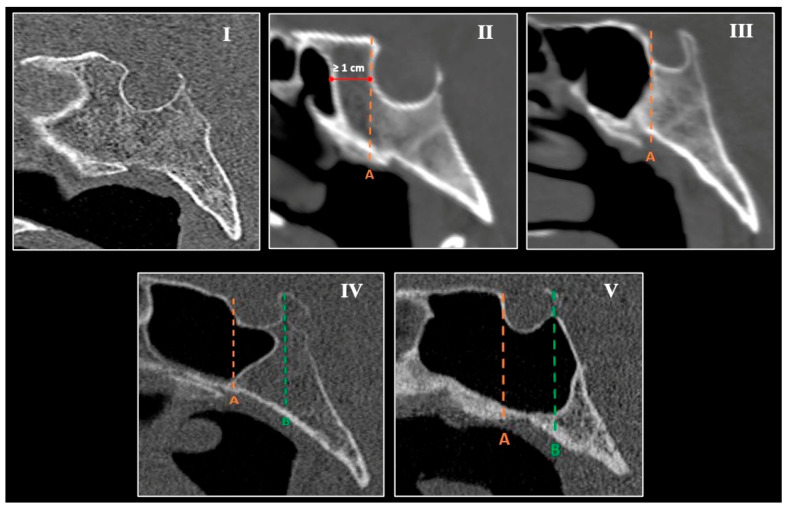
Main types of sphenoid sinus pneumatization (SSP) on midsagittal computed tomography (CT) images. Representative midsagittal CT sections demonstrating the five principal morphologic variants of the sphenoid sinus (SS) based on the relationship of pneumatization to the sella: (I) agenesis—complete absence of sinus cavity; (II) conchal type—minimal pneumatization not extending to the anterior wall of the sella (≥1 cm anterior to it); this case derived from the excluded population and it was not observed in the current sample; (III) presellar type—pneumatization reaches but does not pass the anterior wall of the sella; (IV) sellar incomplete type—pneumatization extends to the posterior clinoid process, involving both the anterior wall and sellar floor; (V) sellar complete type—pneumatization extends beyond the posterior wall of the sella into the basilar portion of the occipital bone. Dotted orange line (A) = vertical coronal plane through the anterior sellar wall; dotted green line (B) = plane through the posterior sellar wall.
